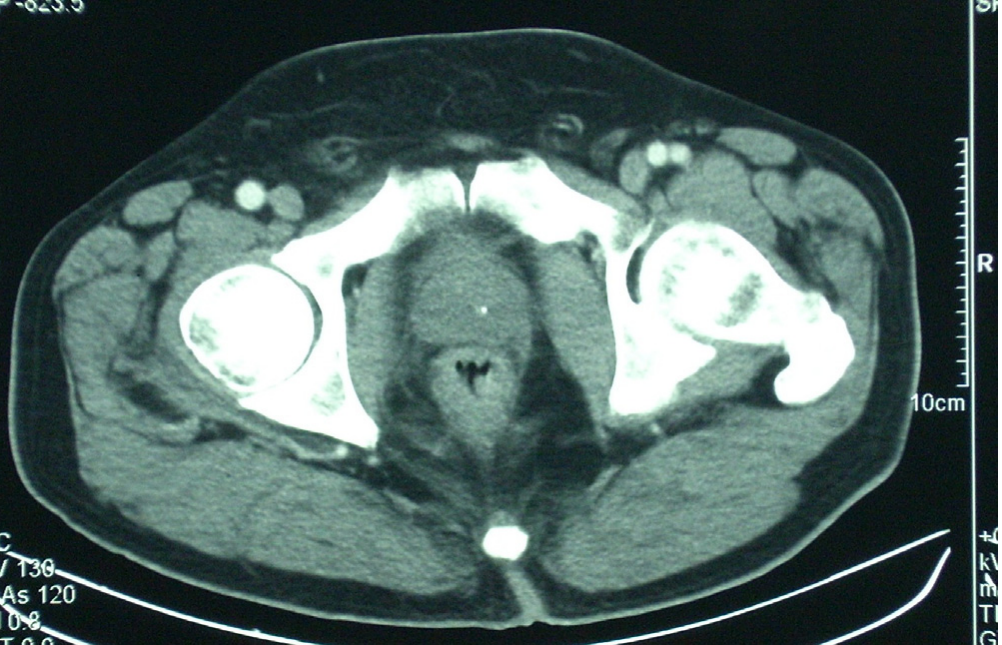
CT at diagnosis showing an eccentric thickening of the rectal wall, compatible with a rectal cancer with no lymph node or visceral involvement.
